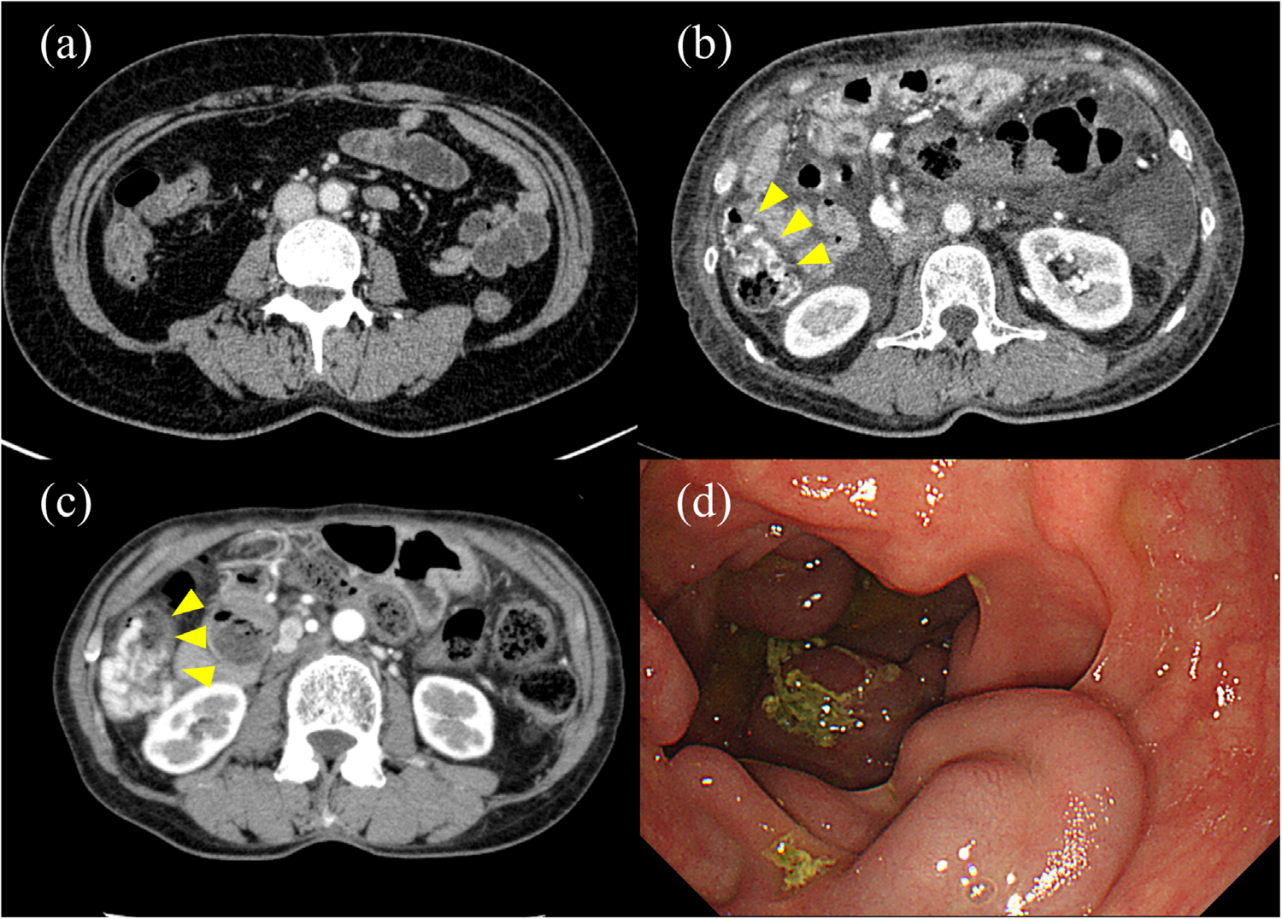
Imaging findings before variceal rupture. (a–c) Contrast‐enhanced computed tomography scan of the abdomen: (a) 6 months after surgery, (b) 1 year after surgery, and (c) 11 years after surgery (January 2022). With the development of collateral vessels, vasodilatation around the ascending colon and vascular structures on the mucosal surface were apparent (yellow arrowhead). (d) Colonoscopy revealed F3‐equivalent tortuous varices, but no red color signs.
